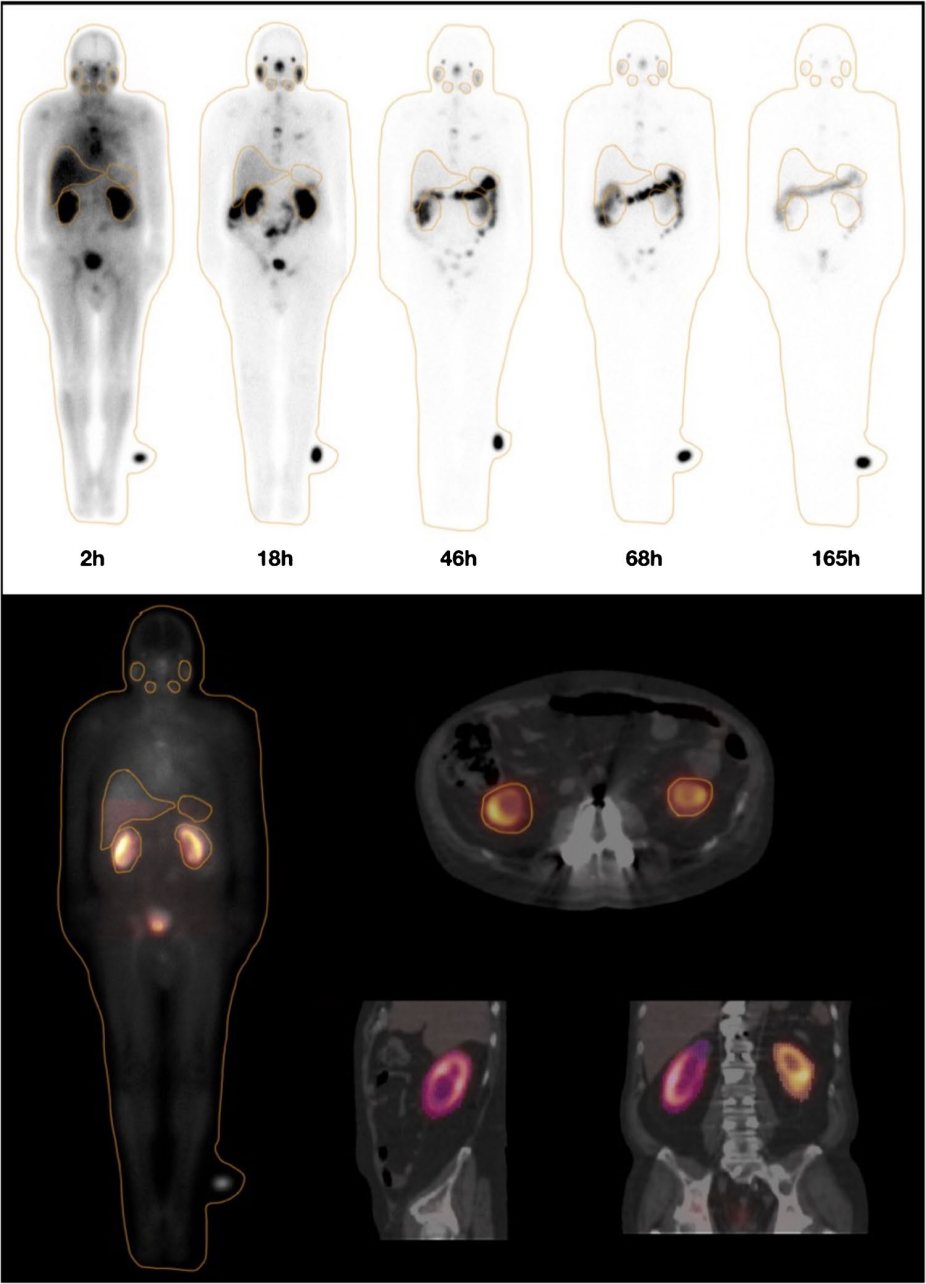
Planar whole-body images of five time points as well as one of the SPECT/CT images. Regions of interest were labeled on the liver, kidneys, spleen, parotid glands, submandibular glands, lacrimal glands, and bladder
